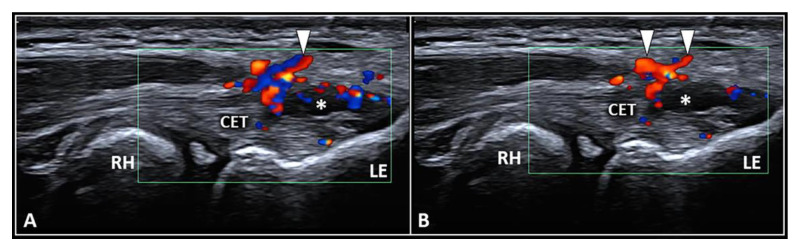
Ultrasound examination with color Doppler (A,B) clearly shows how the aberrant neovessels (*white arrowheads*) originating from the superficial vascular plexus of the subcutis pierce the forearm fascia invading the hypoechoic zone (*white asterisk*) of the CET related to insertional tendinosis. RH: radial head, LE: lateral epicondyle, CET: common extensor tendon.
